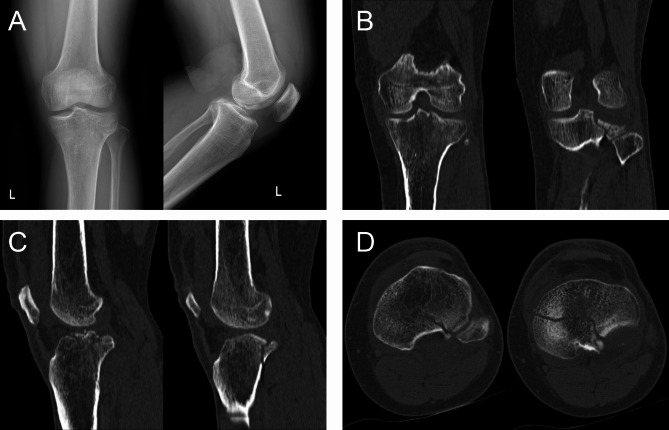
The patient’s preoperative radiological characteristics of PTPF. A. The anteroposterior and lateral X-ray. B, C, D. The coronal, sagittal and transverse CT images of PTPF
